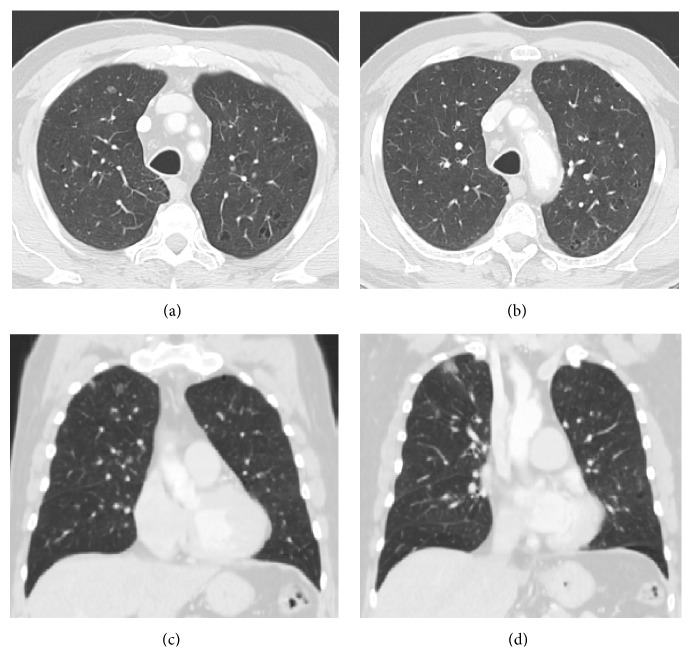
Selected axial and coronal HRCT images on lung windows show bilateral pulmonary cysts. Some of them are rounded and some demonstrate bizarre shapes with multiloculation and internal septations. There are also bilateral ground-glass nodules ranging from 1-2 mm up to a few centimeters. The findings are upper lobe predominant and spare the lung bases.
